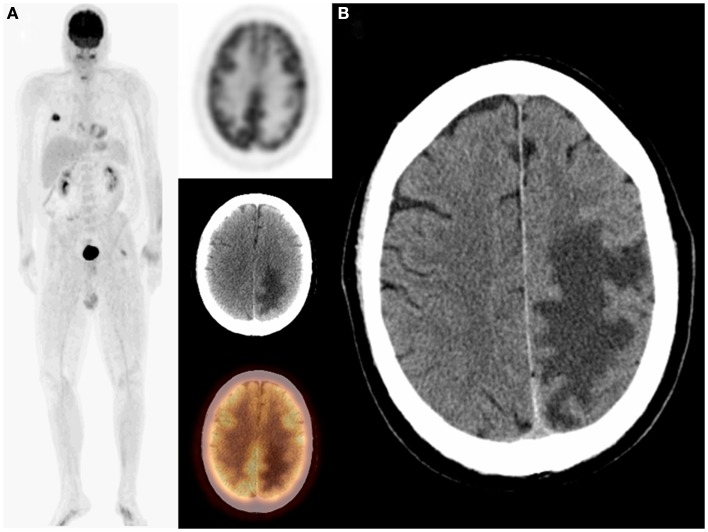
Sixty-five-year-old female with a history of thyroid and metastatic breast cancers as well as schwannoma which was surgically resected. On restaging PET/CT: MIP, transaxial PET, CT, and fused images (A) demonstrated an unsuspected finding of recurrent schwannoma in addition to metastatic breast cancer. PET/CT finding was confirmed on MRI (B). The patient subsequently underwent surgical resection of the recurrent shwannoma.
